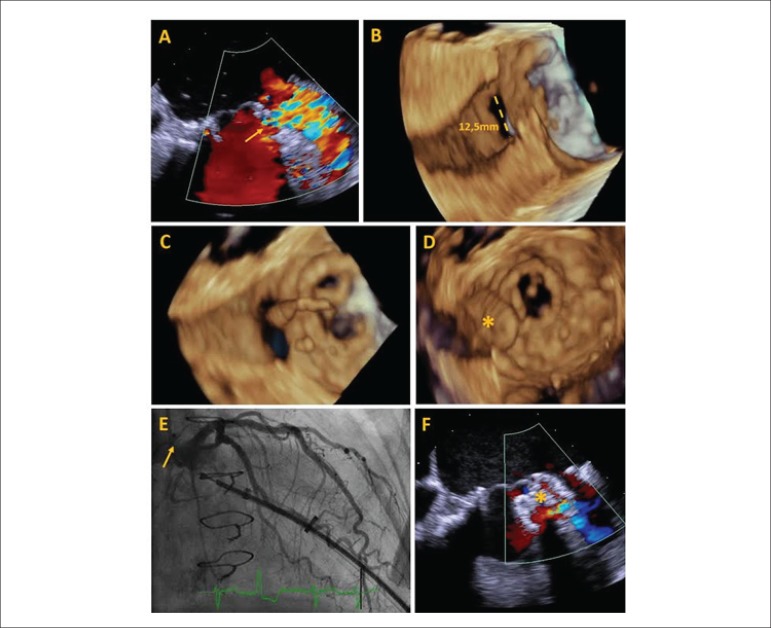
Panel A: 2D peri-processual transesophageal echocardiography (TEE) shows
